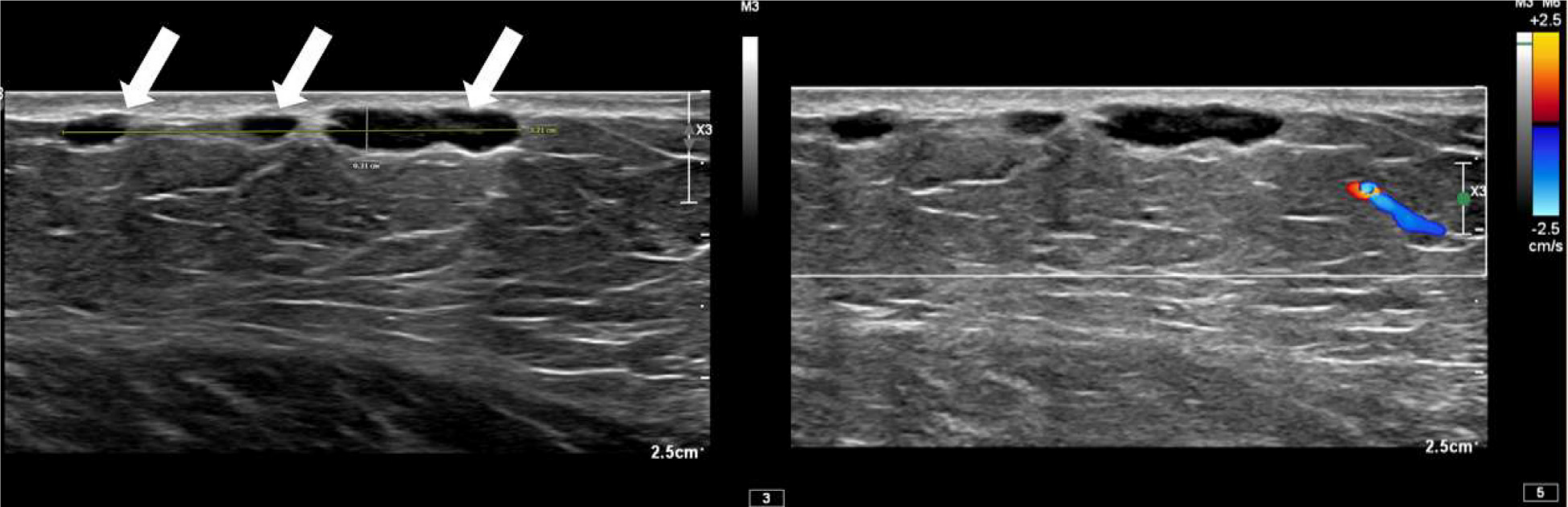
Transverse gray scale ultrasound of the area of palpable concern in the left low axilla demonstrates lobulated, circumscribed subcutaneous masses without internal vascularity, collectively measuring 32 × 3 × 9 mm.
